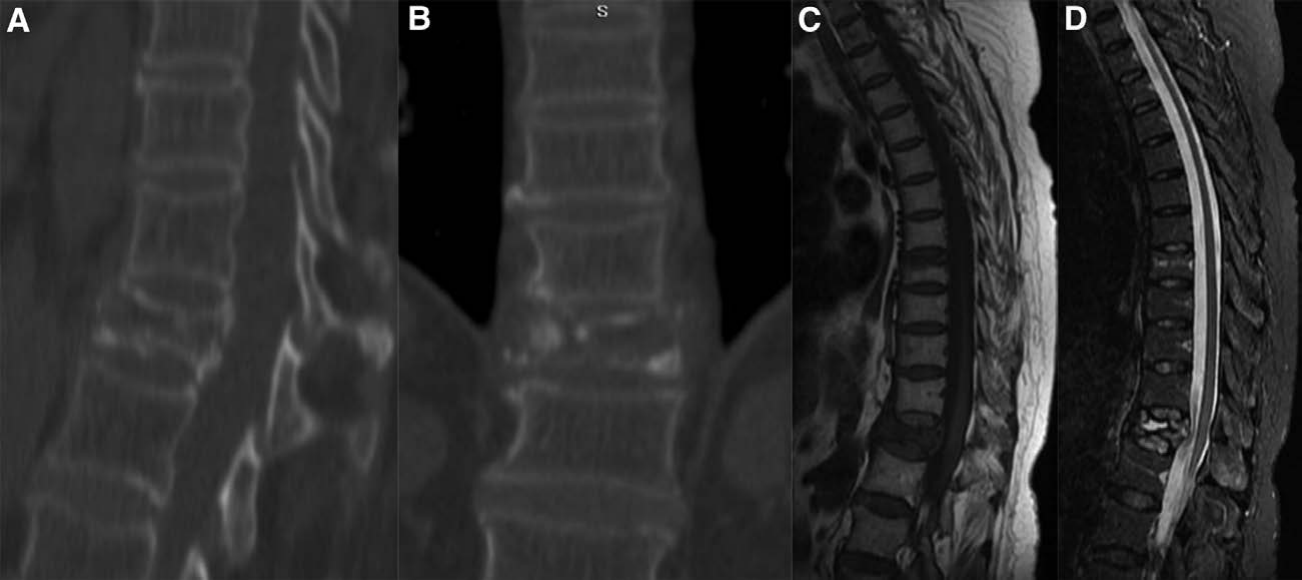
The images for T12 and T8 vertebral bodies. A, B: Axial (A) and coronal (B) CT scans confirmed the severe collapse together with a linear intervertebral vacuum cleft of T12 at the time of admission. C, D: Axial T1-weighted (C) and STIR (D) MR images showed an osteoporotic compression fracture of T8 and a linear intervertebral vacuum cleft in T12. CT = computer tomography.
